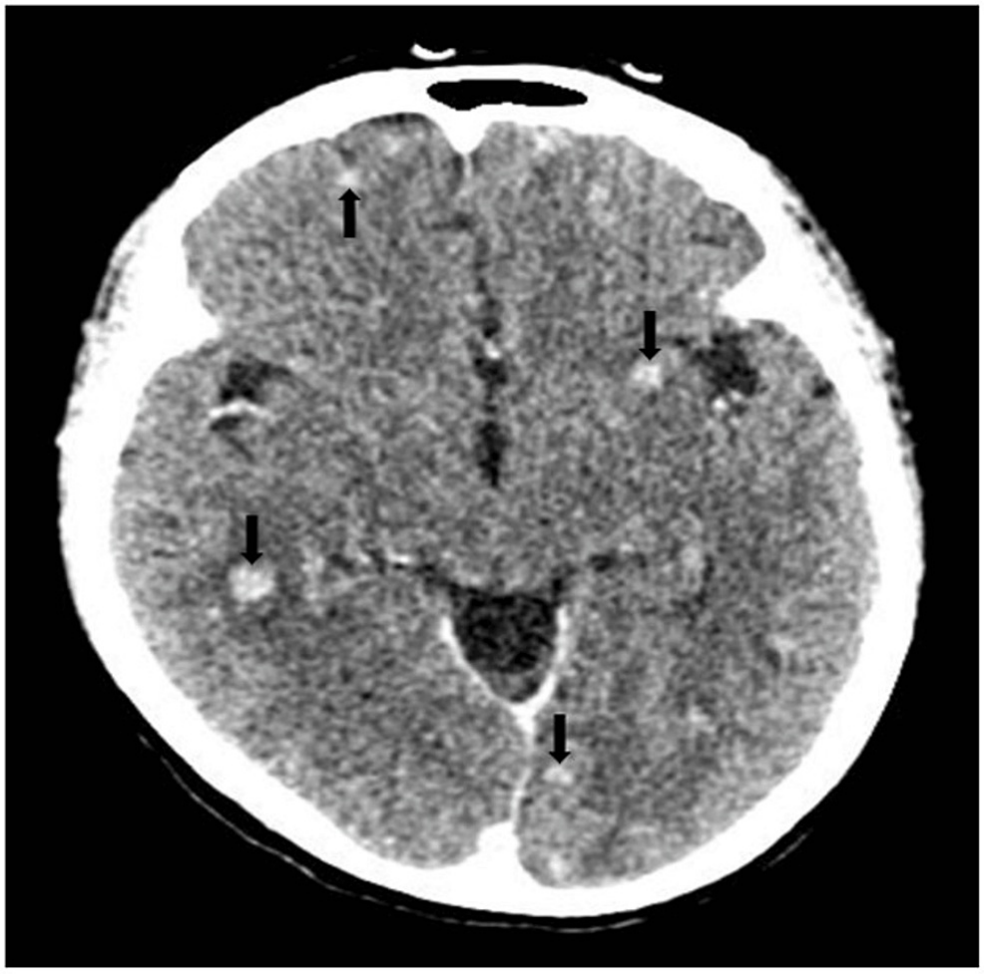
Axial CECT scan Axial CECT scan of the brain showing multiple tuberculomas (marked by black arrows) with variable surrounding white matter edema in a TBM patient. CECT: contrast-enhanced computed tomography; TBM: tuberculous meningitis
